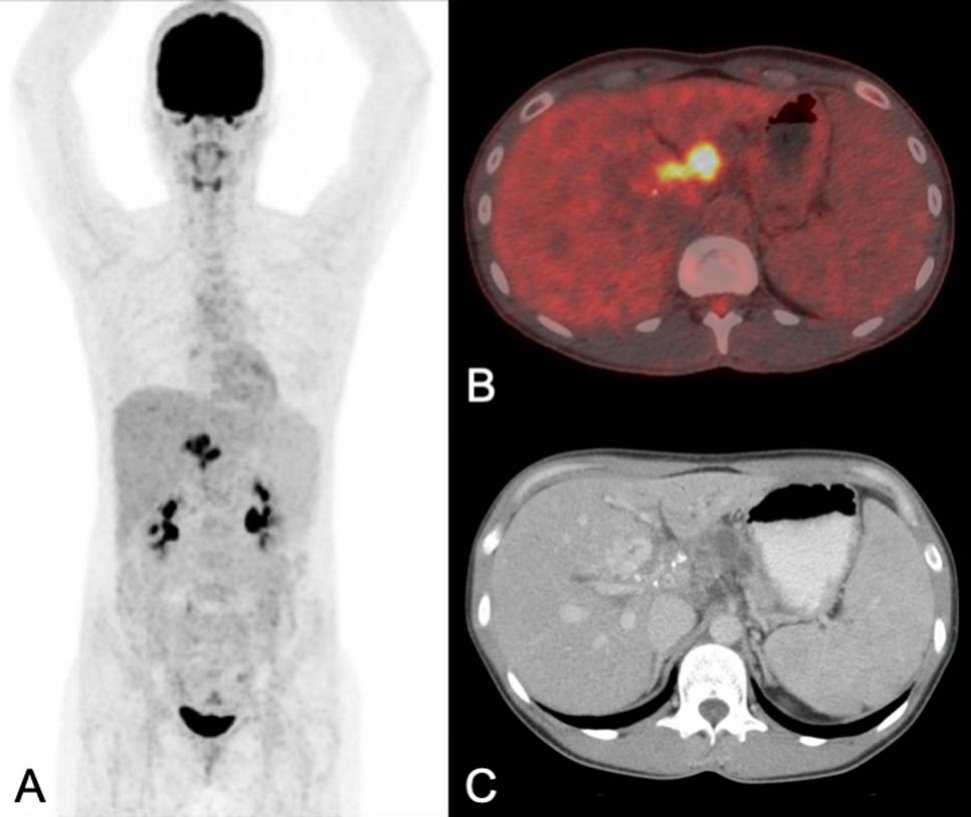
PET/CT performed for staging of alveolar echinococcosis in a 37-year old woman (patient 23 in Table 3) showed intense metabolic activity [in maximum intensity reconstructions of PET (A) and fused PET/CT images (B)] in a hilar lesion infiltrating the pancreas (dilated pancreatic duct on axial contrast-enhanced CT images in (C). SUVmax was 11.1 in the echinococcosis manifestation, and 3.1 in non-infected liver tissue; SUVratio was 3.6. After 1458 days of benzimidazole therapy, levels of Em-18 antibodies became undetectable, but therapy was not yet stopped at the end of the present study period (in total after 1633 days of therapy).
